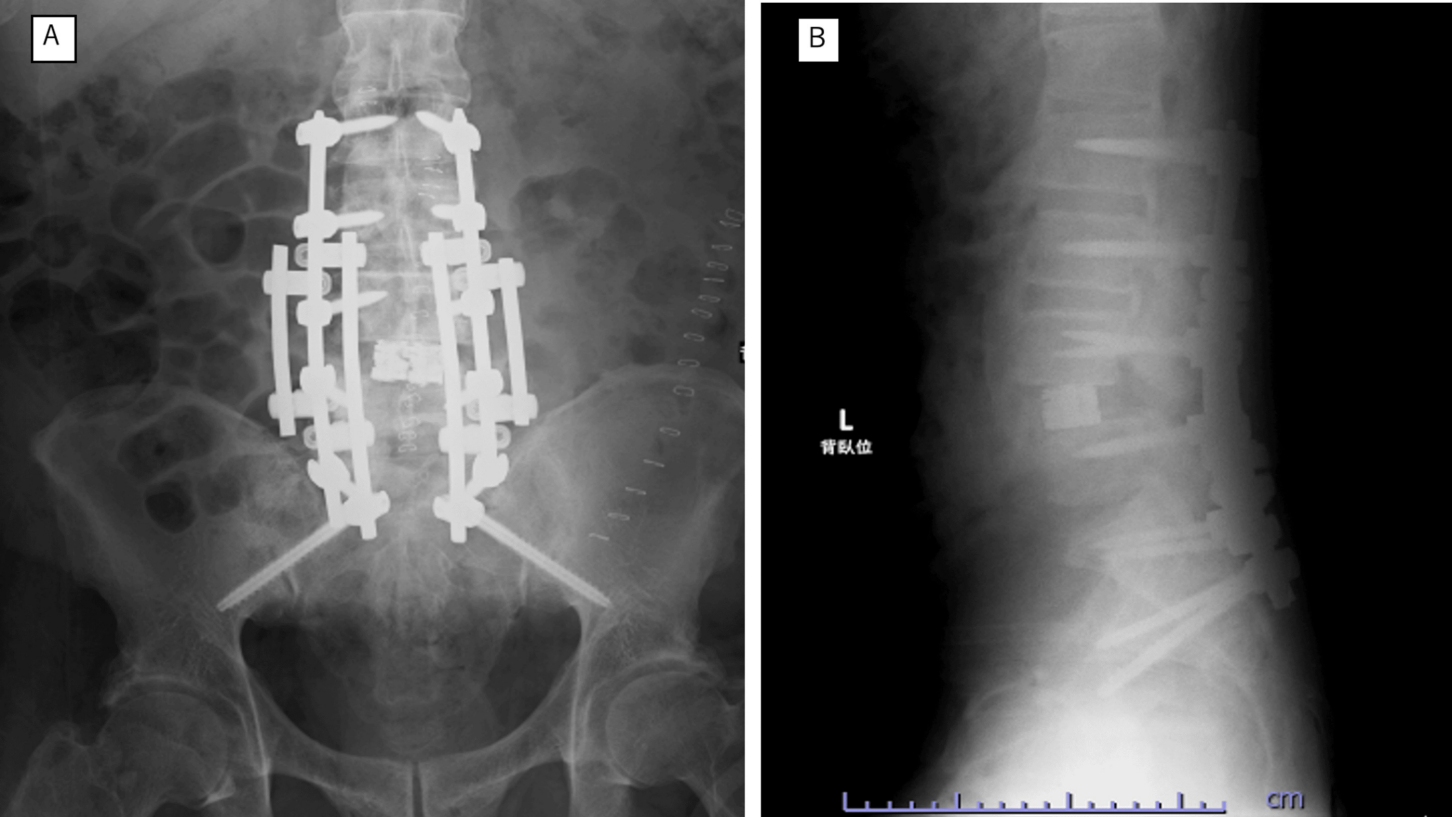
Postoperative radiographs after staged reconstruction. (A) Anteroposterior radiograph. (B) Lateral radiograph. Long-segment posterior fixation with pelvic anchorage and anterior reconstruction at L4/L5 achieved restoration of alignment and stabilization of the unstable segment.
